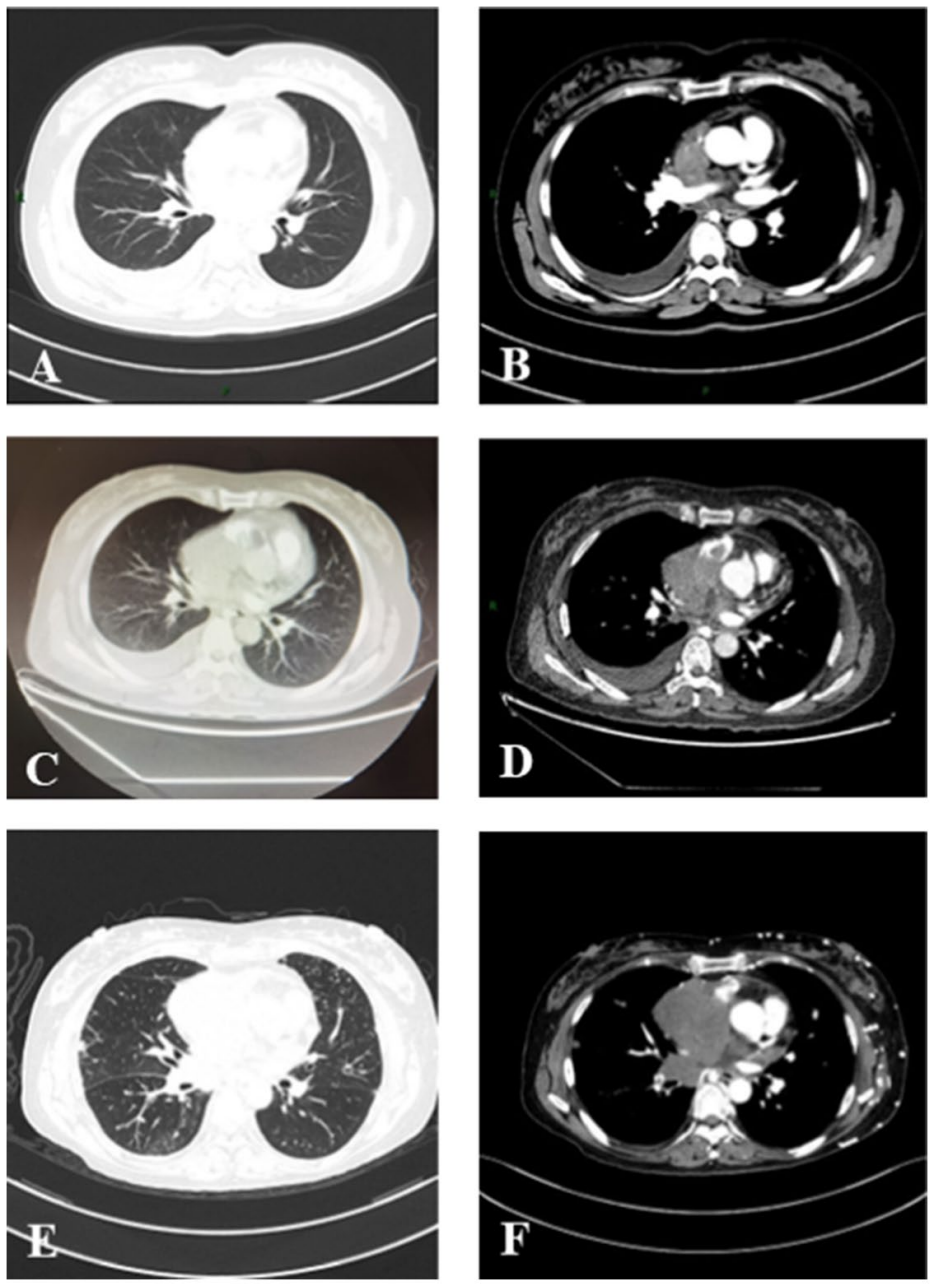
Serial chest CT scans of a patient with PCSS. (A,B) The initial CT scan on 14 December 2020 showed an irregular mass in the vena cava and right atrium with massive right pleural effusion. (C,D) A follow-up CT scan (15 January 2021) showed an enlarged mass measuring 4.0 × 3.4 × 7.6 cm in size in the vena cava and right atrium with pleural effusion on the right side. (E,F) CT scan on 8 March 2021 showed a rapidly enlarged mass in the vena cava and right atrium.
